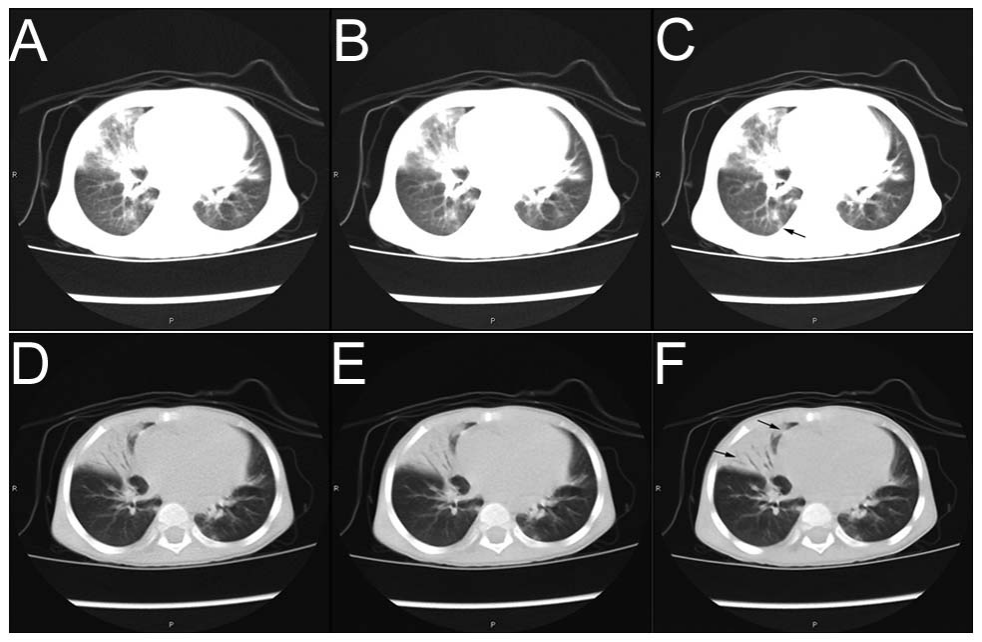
A 2-year old girl with fever for 15 days and cough for 2 days. X-ray suggested severe pneumonia, and enhanced CT scanning was performed for further examination. Tube voltage was 120–13 mA. CT revealed large areas of consolidation and air bronchogram, and the girl was diagnosed with adenovirus pneumonia. CT images displayed here were reconstructed with FBP (1-A), ASIR (1-B), and MBIR (1-C). The tube current of the presented layer was 13 mA, the window level was −700, and the window width was 1000. The MBIR image had the lowest noise in the three images, which displays the lung field and the region of the lesions clearly. Arrow shows the lesion is clearly displayed. Images were reconstructed with FBP (1-D), ASIR (1-E), and MBIR (1-F). The tube current of the presented layer was 13 mA, the window level was -450, and the window width was 1500. The MBIR image had the lowest noise among all three images. The lower arrow shows where that the air bronchogram is clearly displayed, while the upper arrow shows the air bronchogram that was not displayed in the other two images
